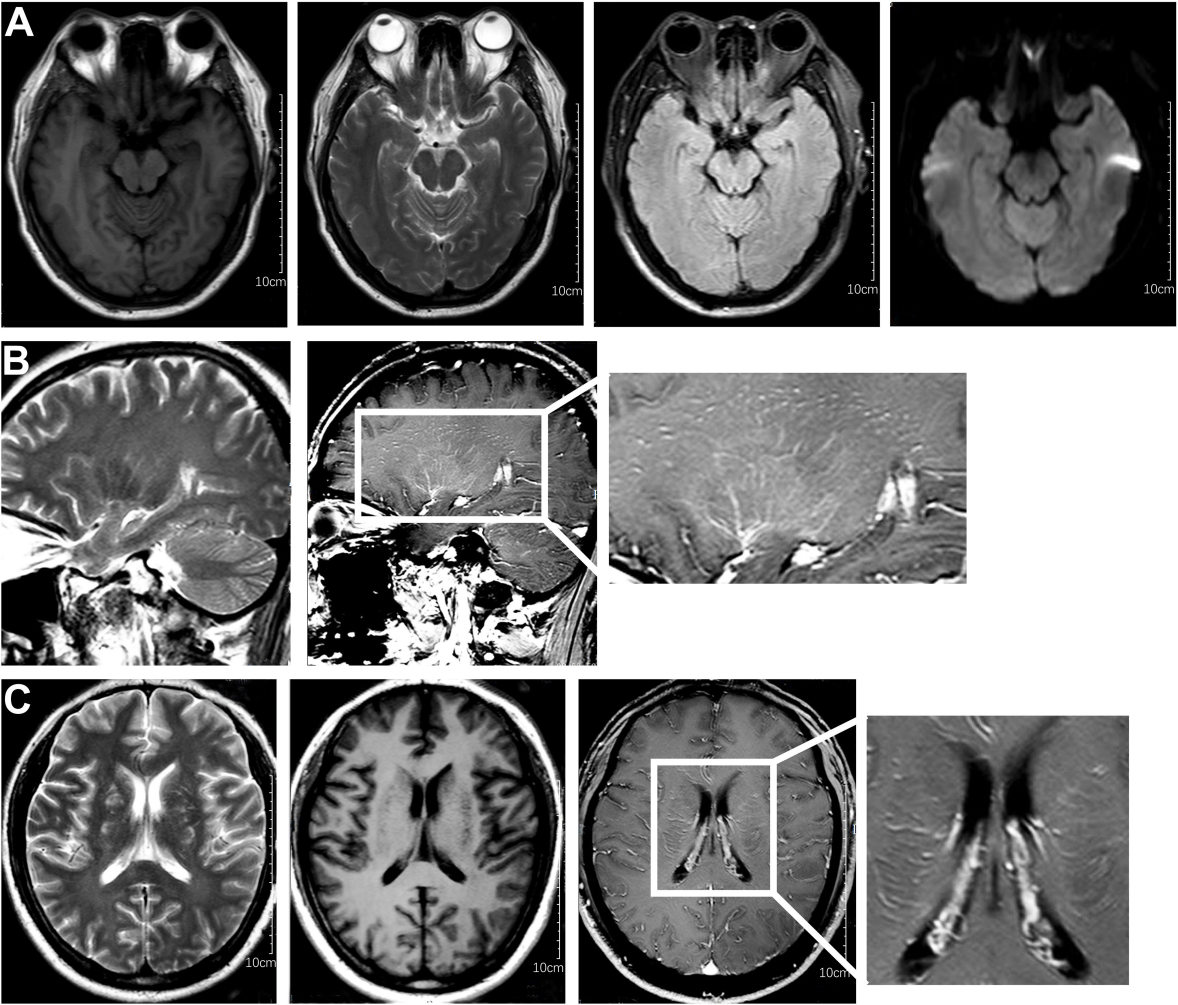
Characteristic brain MR images of autoimmune GFAP astrocytopathy. (A) Brain MRI showed the disappearance of T2/flair high signal in the cerebral cortex and bilateral hippocampus compared with previous brain MRI. (B) The sagittal section showed linear perivascular radial gadolinium enhancement in the white matter perpendicular to the ventricle. (C) Brain MR axial position suggests vascular enhancement perpendicular to the lateral ventricle.
